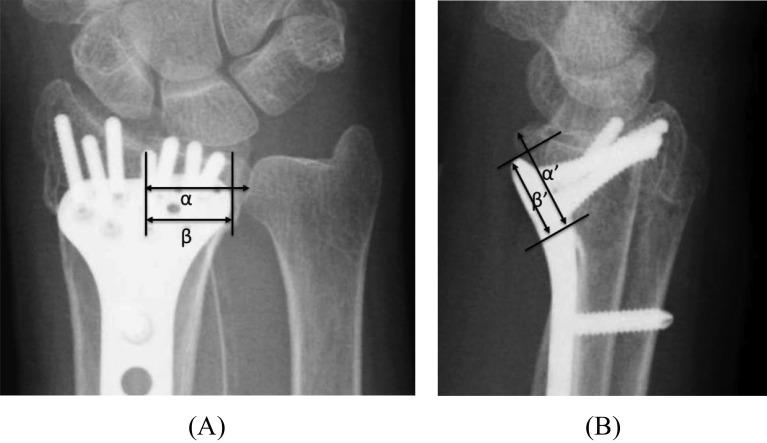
Plate coverage against the transverse dimension of the VLF was calculated as the percentage of the area covered by the plate in the transverse dimension of the VLF on AP radiographs (β/α) (2A). In the same way, plate coverage against the longitudinal dimension of the VLF was calculated as the percentage of the area covered by plate in the longitudinal dimension of the VLF on lateral radiographs (β’/α’) (2B).
